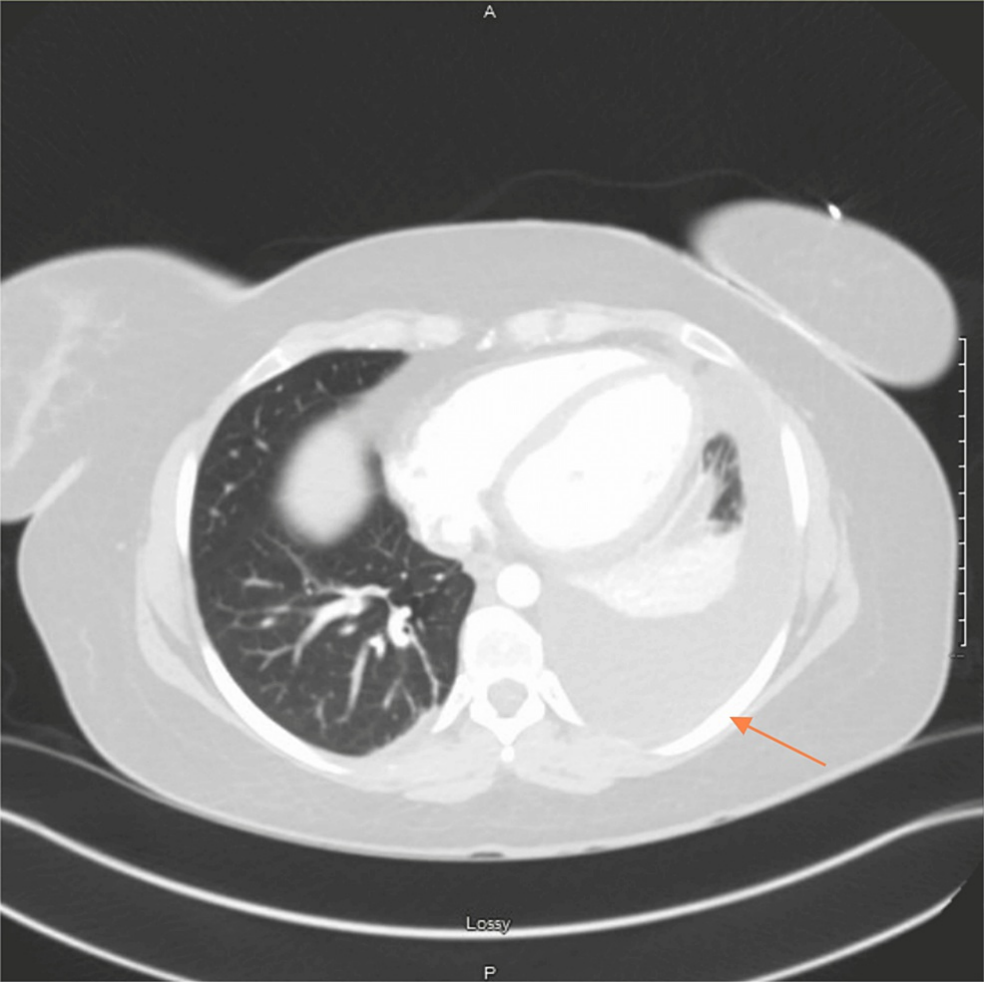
CT angiogram pulmonary embolism (PE) per protocol The orange arrow indicates worsened left-sided pleural effusion.
